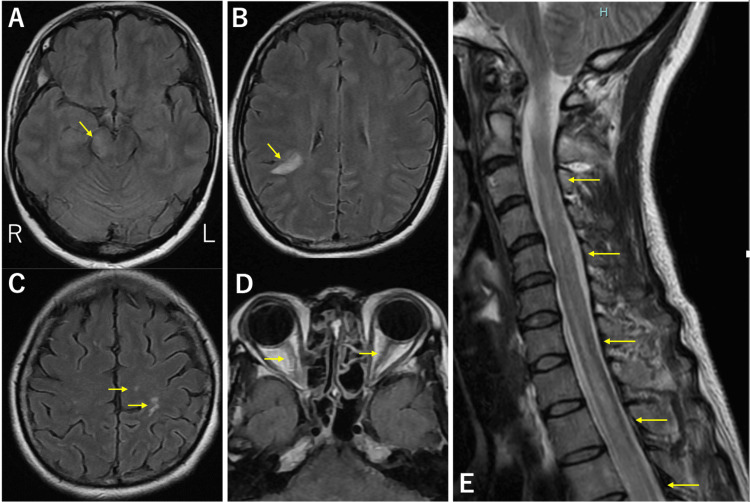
MRI of the brain and spinal cord FLAIR images show high signal intensity in the right lateral midbrain (arrow, A: axial, 1.5T) and bilateral subcortical areas (arrows, B, C: axial, 1.5T). No obvious bilateral abnormalities were observed in the optic nerves (arrows, D: axial, 1.5T). Cervical spine T2-weighted images show hyperintensity along the central canal from the C3 to T2 vertebral levels (arrows, E: sagittal, 1.5T). FLAIR, fluid-attenuated inversion recovery; MRI, magnetic resonance imaging
